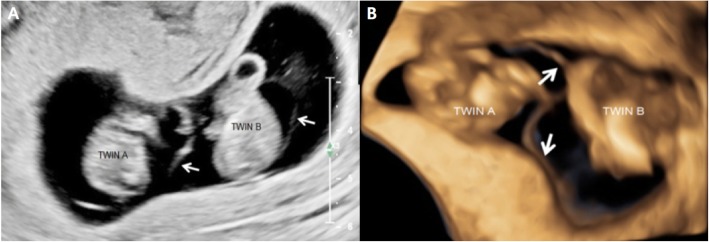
Diamniotic membrane (arrows) between the twins at 9 + 5 weeks in two-dimensional (a) and three-dimensional (b) ultrasonography, respectively
